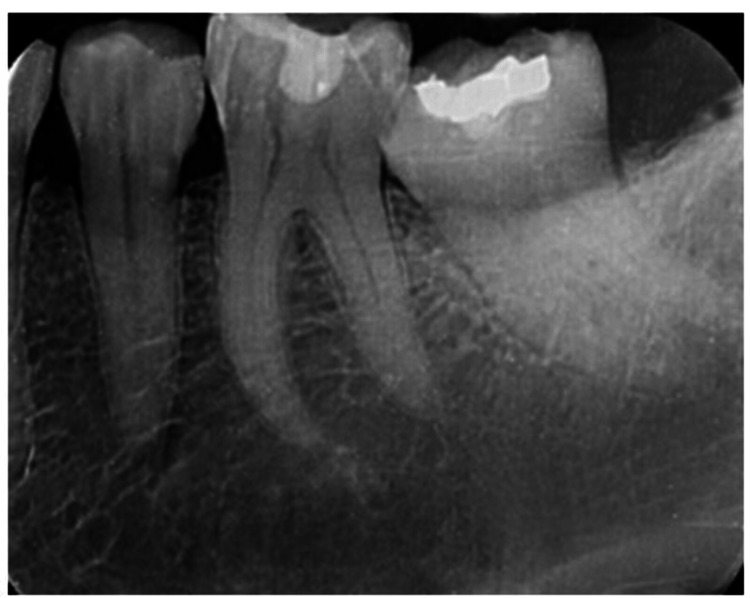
Pre-operative radiograph of 36
